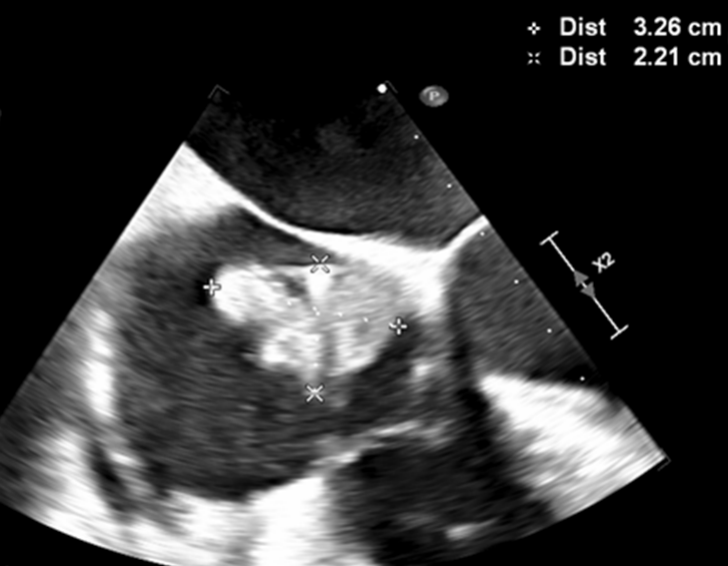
Figure 1. Transesophageal echocardiogram demonstrating size of vegetation.
